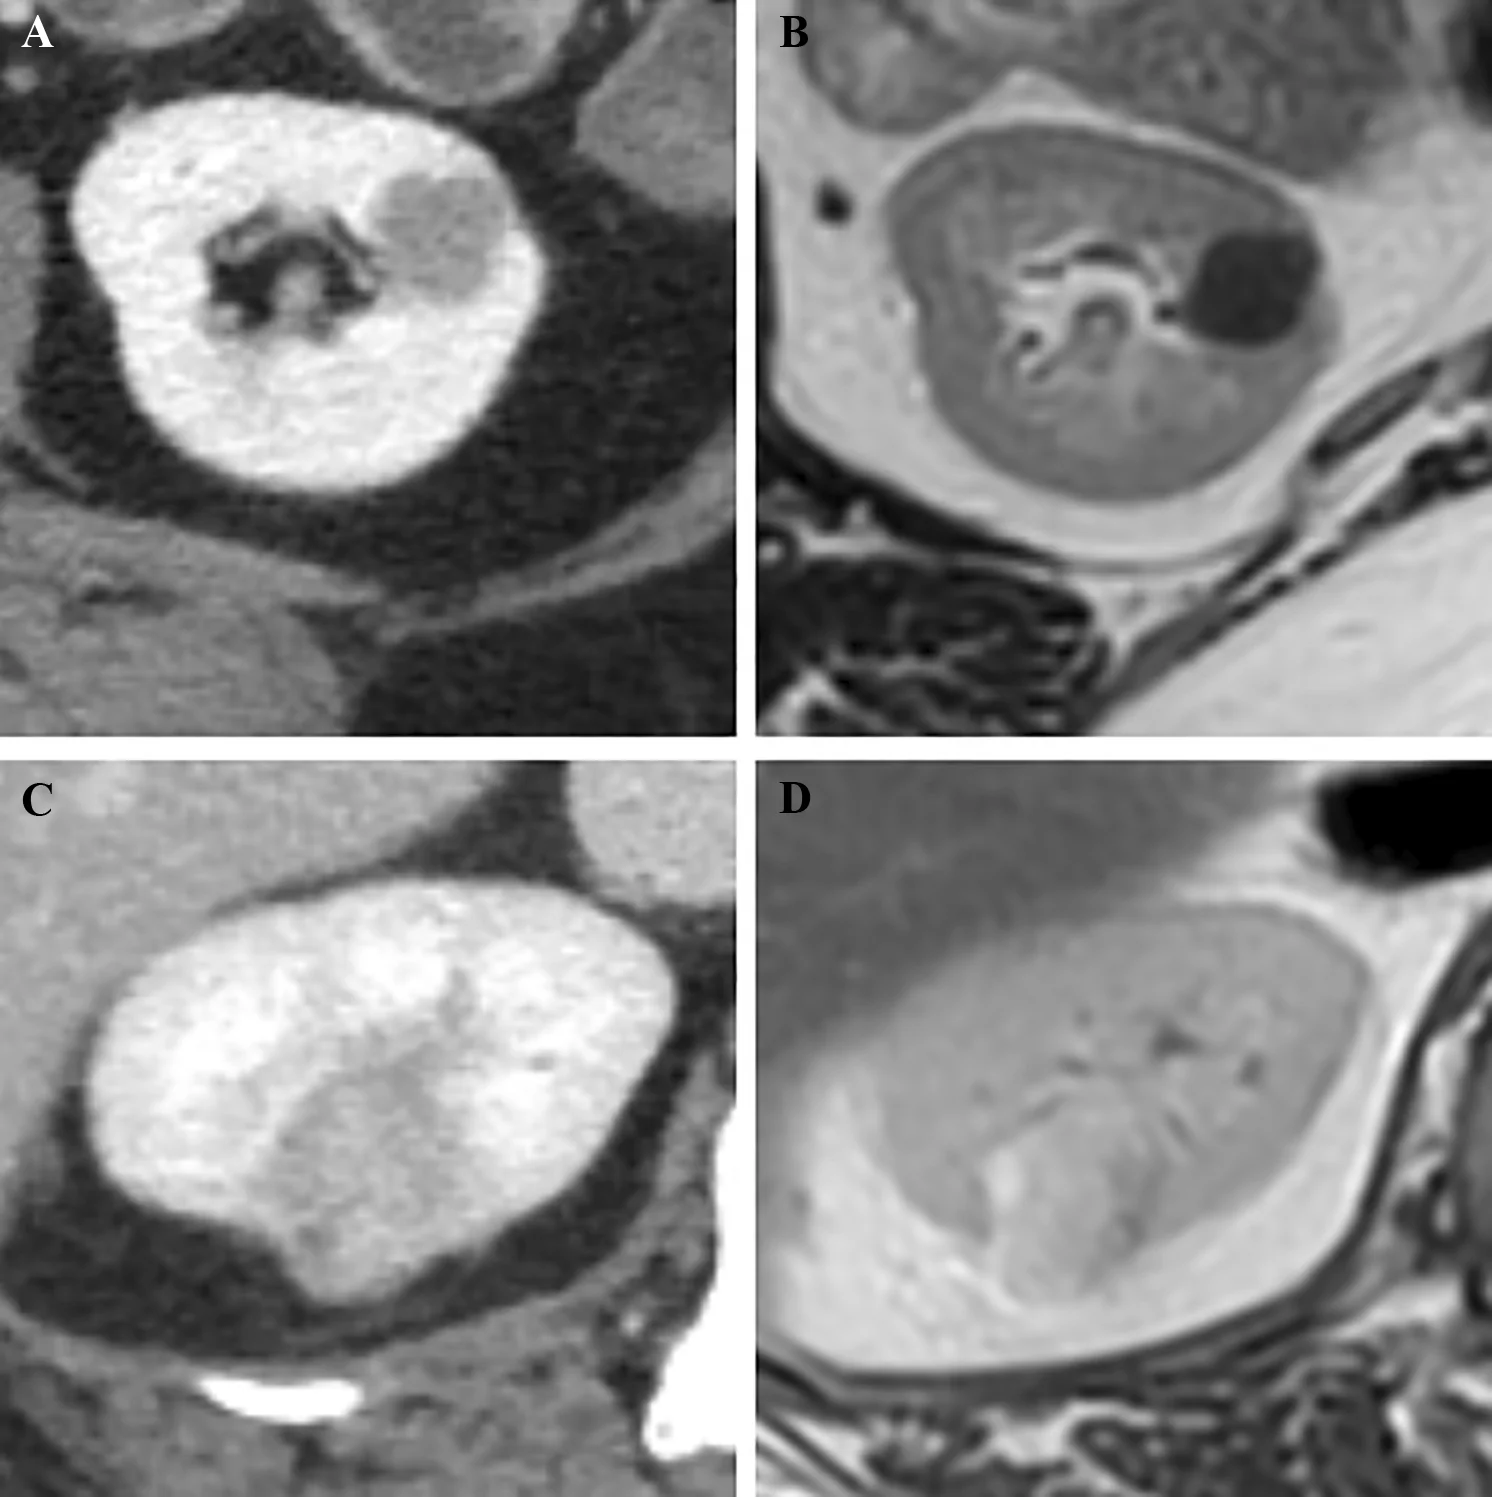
Presents contrast-enhanced CT and T2-weighted images in the axial plane of two small renal masses. A 1.7 cm right-sided, well-circumscribed, endophytic small renal mass in a 70 year-old female patient depicted as homogenous and with a lower degree of enhancement compared to renal parenchyma on contrast-enhanced CT (A) and lower signal intensity relative to renal parenchyma on an MRI T2-sequence (B). A 2.7 cm right-sided, partly exophytic small renal mass in a 65-year-old male patient depicted as slightly heterogeneous with contrast-enhanced CT (C) and with hyperintense signal intensity relative to renal parenchyma on a T2-weighted sequence (D)
